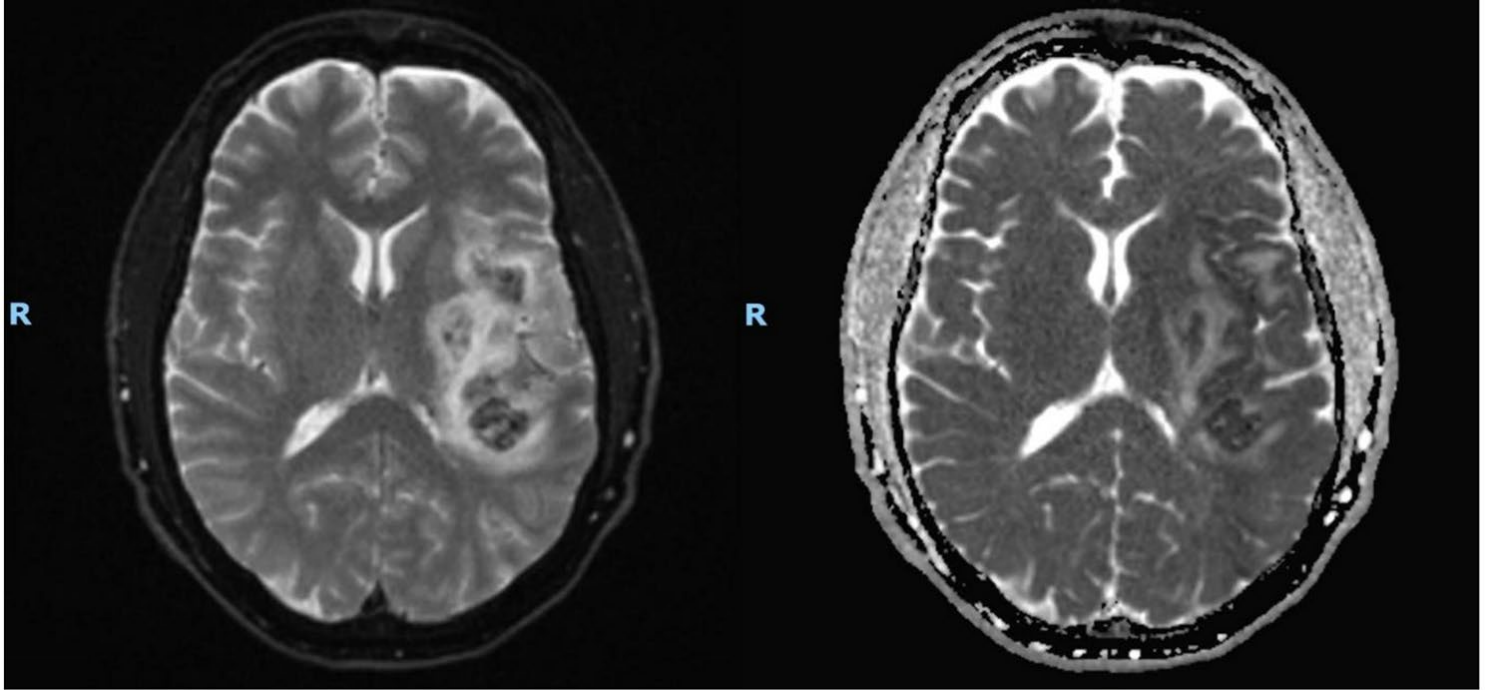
MRI imaging performed 9 days following left MCA territory stroke. Diffusion-weighted imaging (DWI) left and corresponding apparent diffusion coefficient (ADC) right
